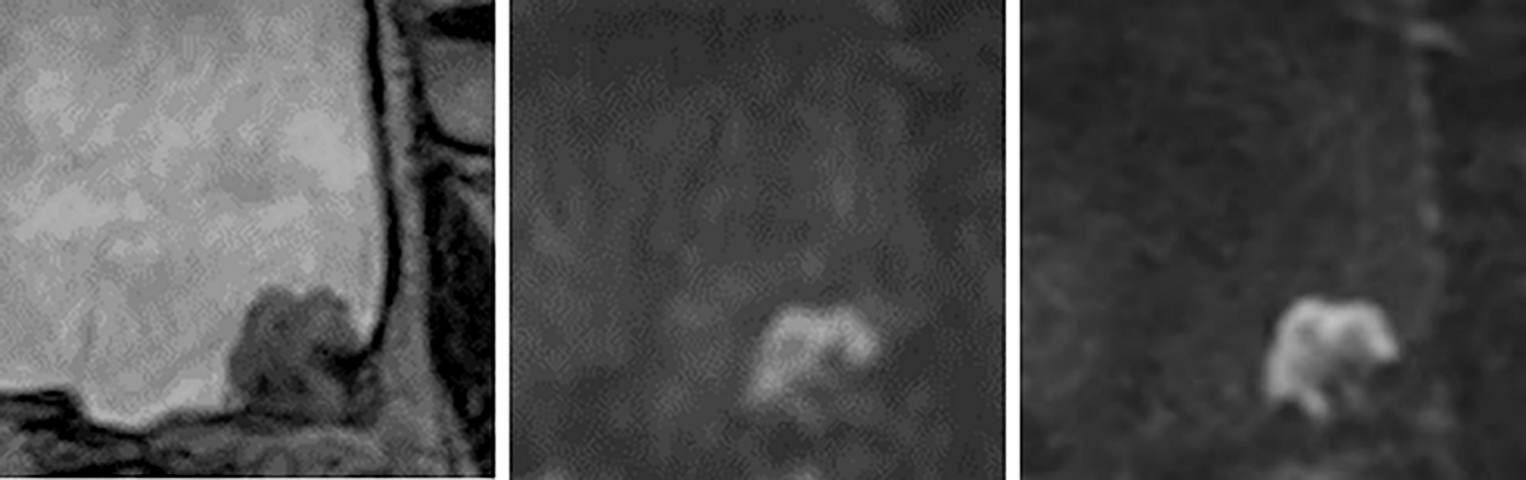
61-year-old woman with the urothelial carcinoma of pTa on the posterior wall of the bladder. (A) T2WI (B) fFOV DWI (C) Zoomed DWI. VI-RADS score was 3 for reader 1 and 2 for reader 2 with fFOV DWI. In contrast, the tumor stalk clearly appeared on Zoomed DWI, and VI-RADS score was 2 for both readers.
